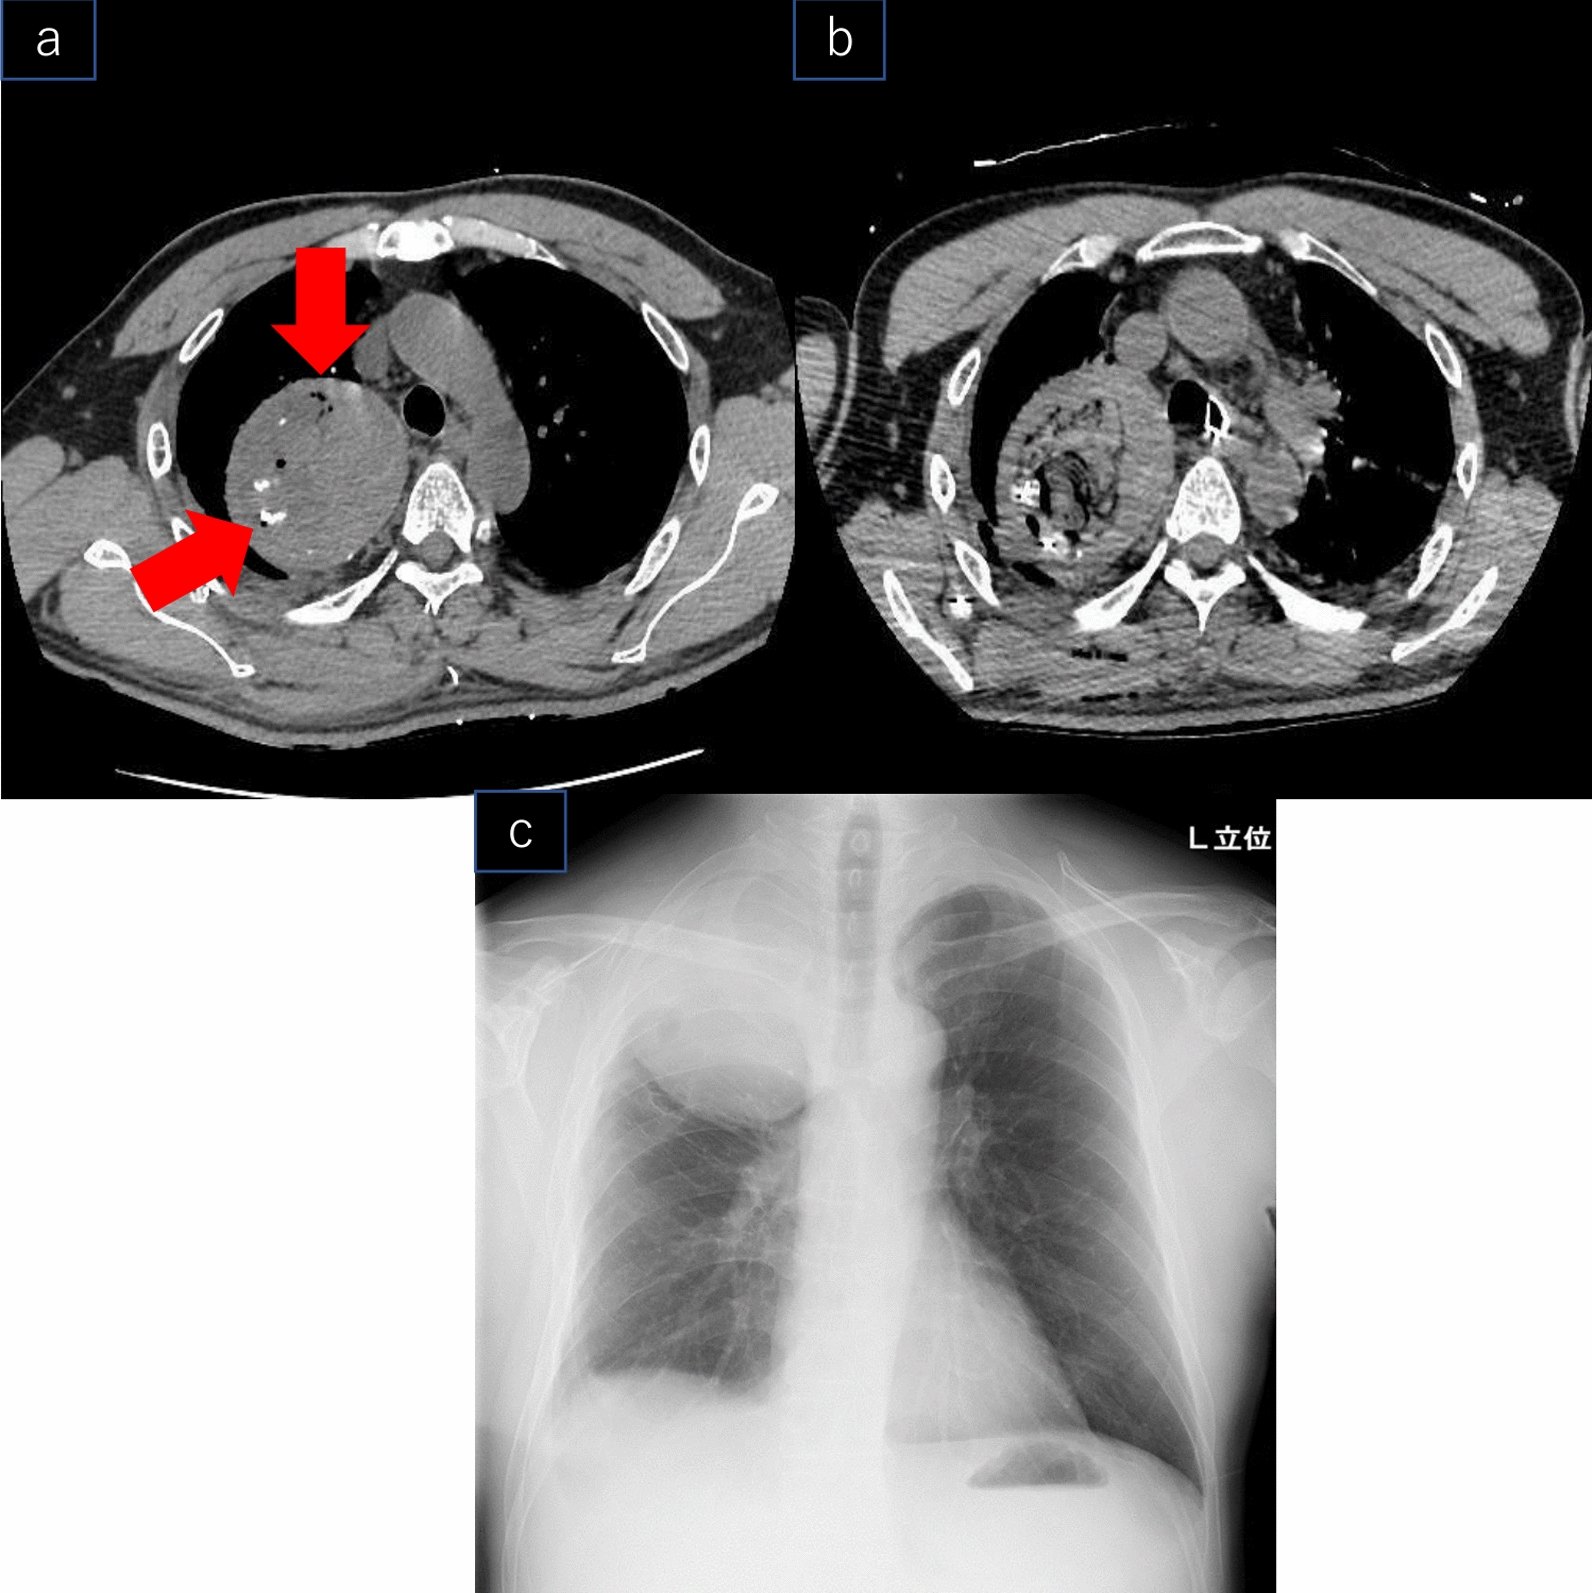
a Arrowhead indicates the non foreign object removal area and gauze. b Computed tomography reveals that the foreign object removal is completed. c X-ray 5 months after the operation
